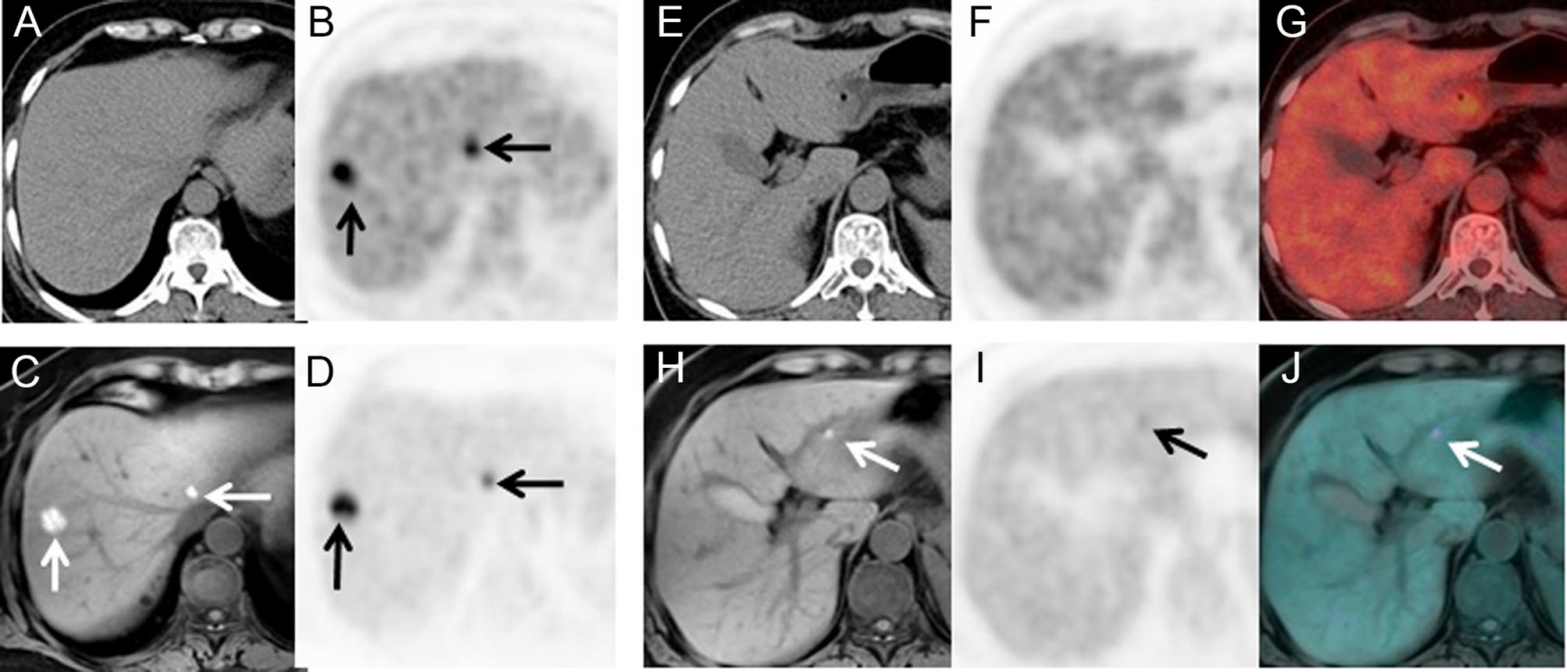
A 66-year-old female with surgical resection of malignant melanoma on head skin. She received PET/CT scans as regular follow-ups. Only two lesions were seen on PET/CT (A, B). No lesion was seen on the left lateral lobe (E–G). PET/MR clearly showed two lesions with hyperintensity on T1WI and hypermetabolism (C, D), and an additional 4-mm small lesion was identified on T1WI and PET (H–J), which only showed mild FDG uptake (T/N = 1.3).
